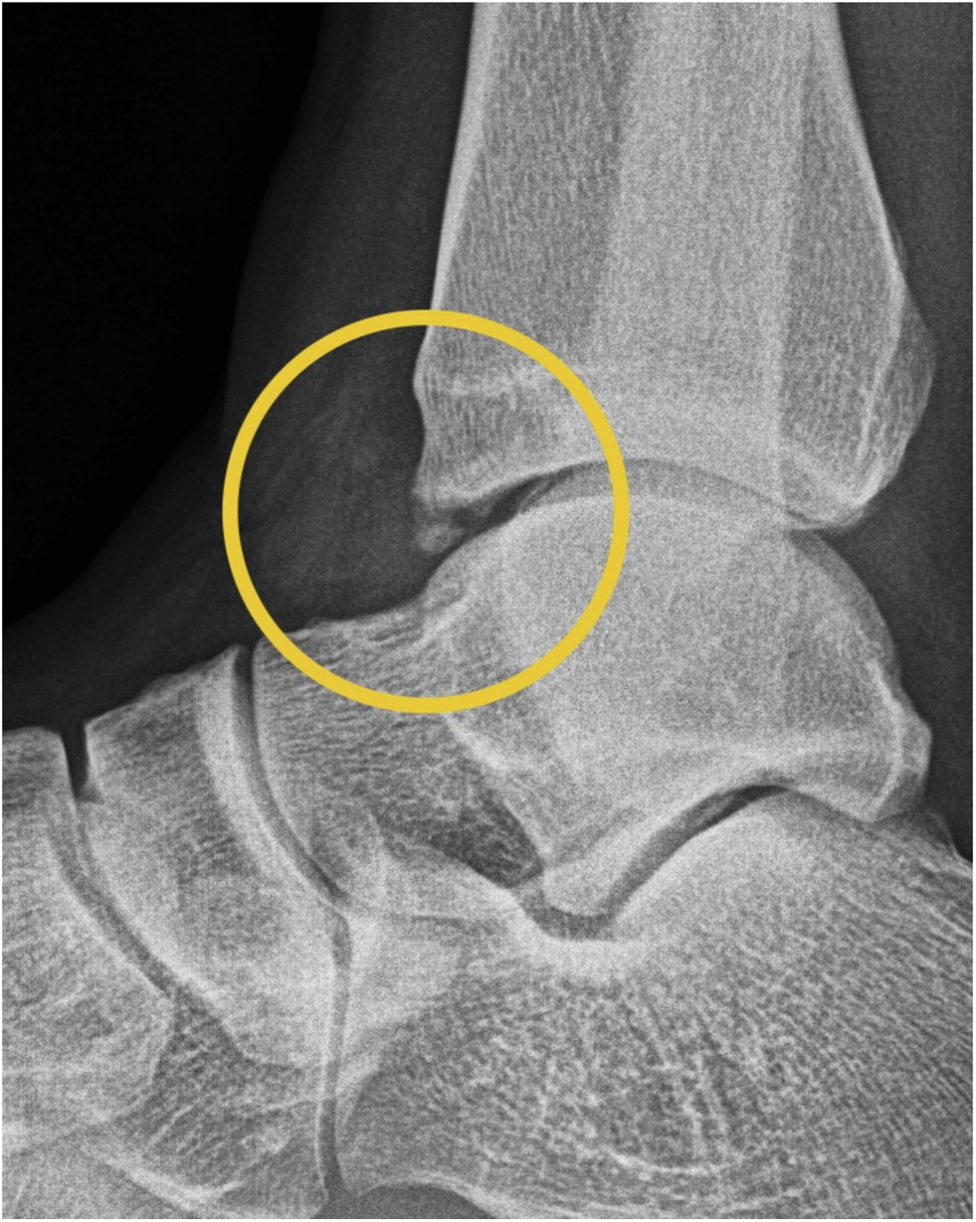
Imaging. Typical findings of radiographic imaging (Scranton and McDermott classification grade 2). Lateral view. Right ankle.
